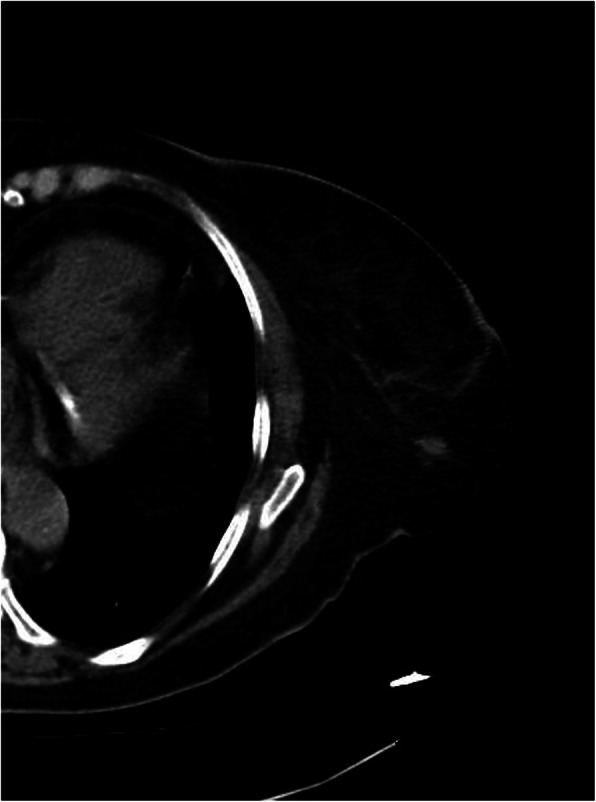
A computed tomography scan revealed nodular opacity under the left nipple and axillary adenopathy
